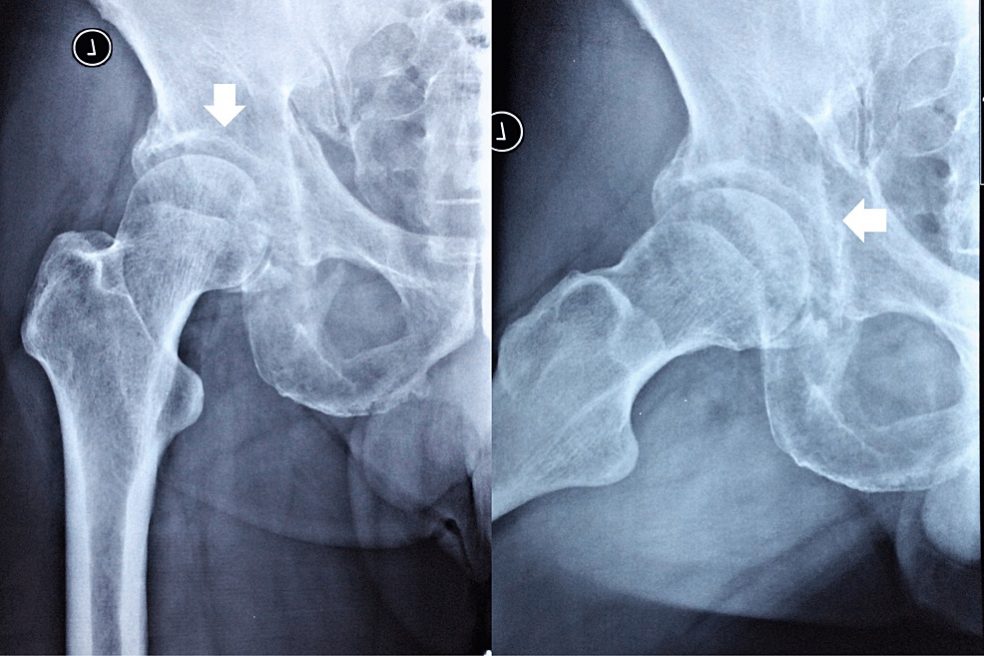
Hip radiography Hip radiographs identified multiple lucent foci on the left pelvic rim, femoral head, and proximal body (white arrows).
